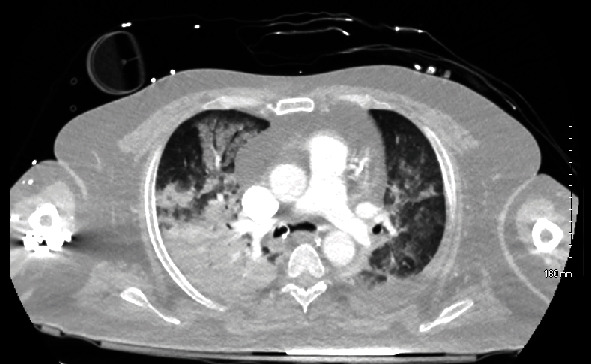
CT angiography chest abdomen pelvis showing extensive, heterogeneous, ground glass, and consolidative opacities throughout the lungs bilaterally, including dominant, dense consolidations in the right upper and right lower lobes. A small left pleural effusion is also noted.
